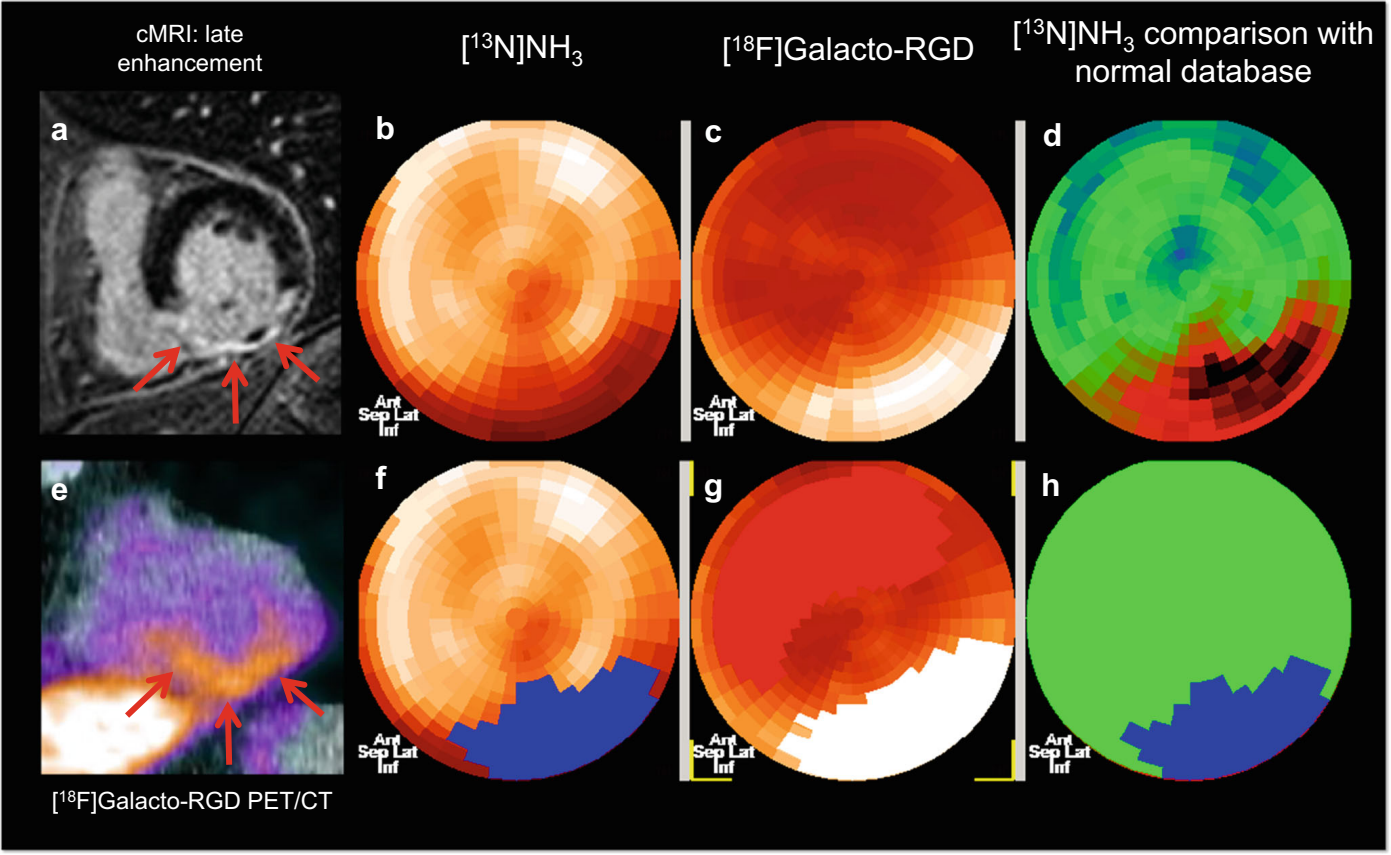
Image analysis in a patient with inferior wall myocardial infarction. Cardiac MRI (A: short axis late enhancement images) shows intense contrast enhancement in the inferior wall with central no reflow phenomenon indicative of a severe transmural infarction (red arrows). [^13^N]NH_3_ PET (B, F: polar maps; D, H: comparison with normal database) shows severely impaired blood flow in the inferior wall, the areas coded in red in D indicate areas with substantially lower flow compared with normal myocardium in a reference population, and the areas in blue in H indicate areas defined as infarcted using a threshold of 0.5 ml/min/g. [^18^F]Galacto-RGD PET/CT (C: polar map without ROIs, G: polar map after manual delineation of the ROI for the infarct area (white) and the remote myocardium without increased [^18^F]Galacto-RGD uptake, with normal perfusion and without late gadolinium enhancement (red); E: short axis PET/CT fusion image) shows pronounced tracer uptake in the area of infarction in the inferior wall with no tracer uptake in normal remote myocardium
